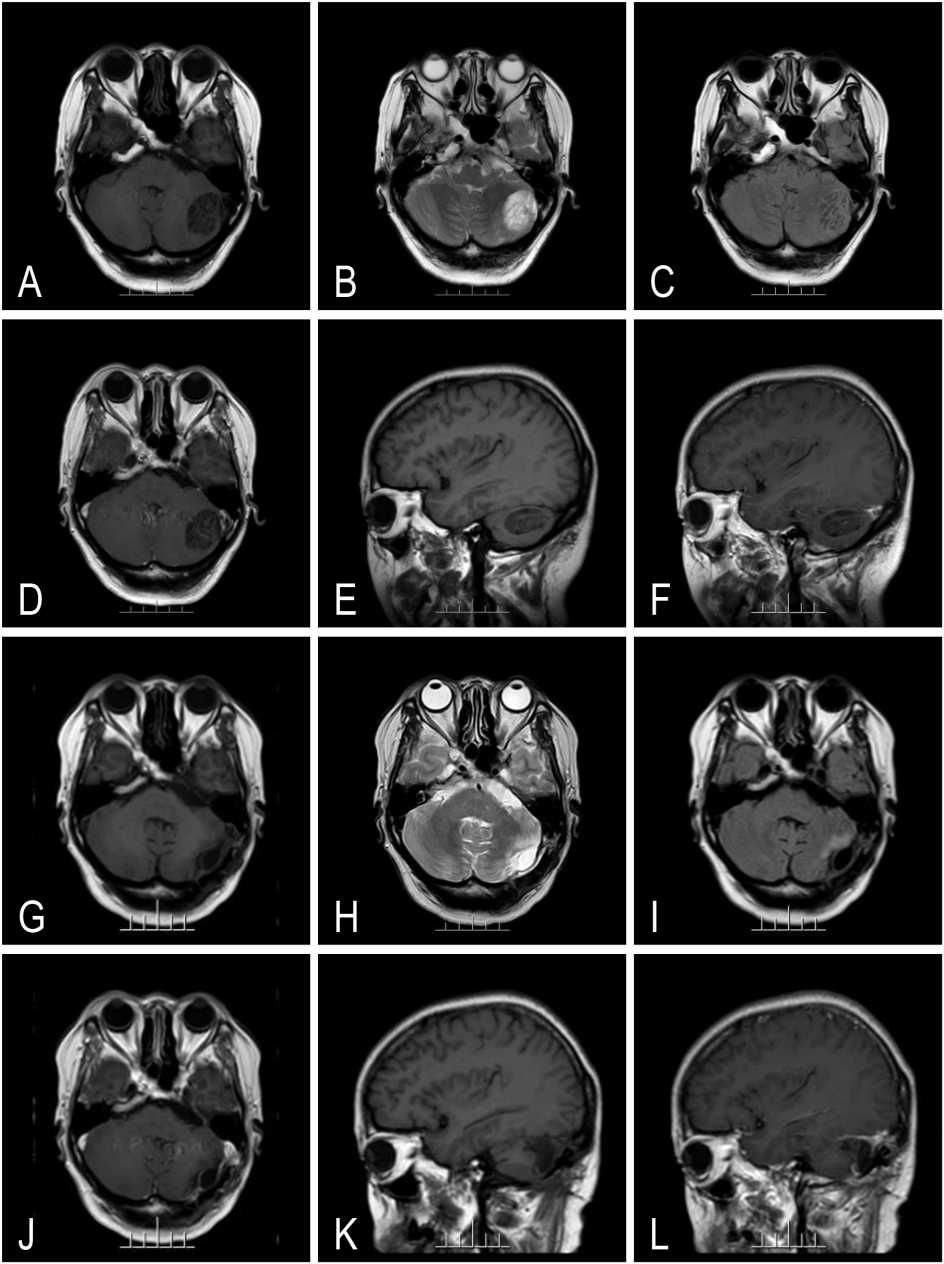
Representative magnetic resonance imaging of case 2 with gross total resection (GTR). It showed a heterogeneous lesion with T1 hypointensity (A) and T2 hyperintensity (B) in the left cerebellum. The lesion shows non-edema (C) and non-contrast enhancement (D) but a striated folial pattern (tiger-striped appearance) (C) in FLAIR or T1-weighted imaging. The tumor was confined to one side of the cerebellar hemisphere according to sagittal projections (E, F) and was completely GTR (G-L).
